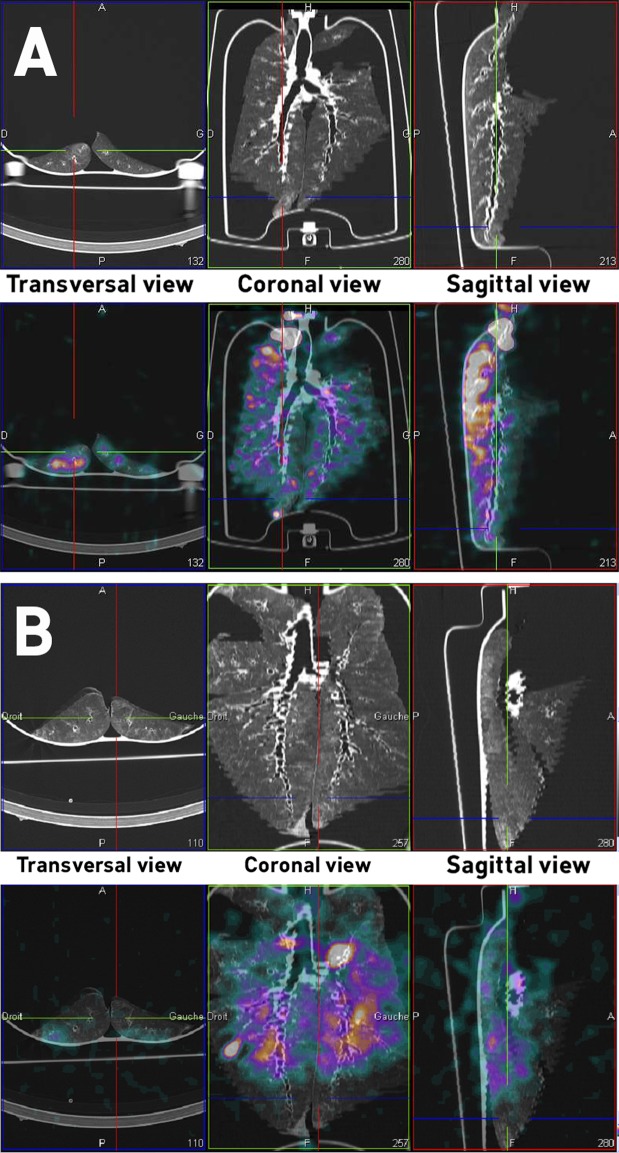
99mtechnetium diethylene triamine pentaacetic acid (^99m^Tc-DTPA) images after three-dimension reconstruction of the *ex vivo* model developed in this study. (A) Images from healthy model. (B) Images from IPF-mimicking lungs. Upper panels: tomography images. Lower panels: fusion of tomography and scintigraphic images.
